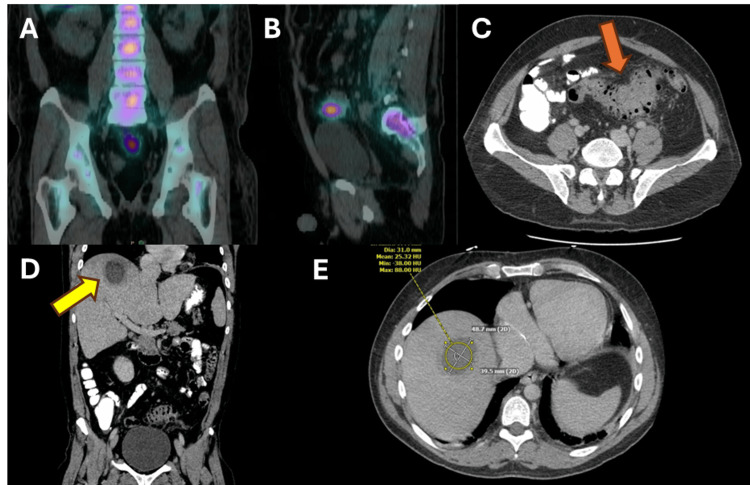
Imaging Studies of the Hepatic Abscess and Diverticulitis Indium-111 radionuclide scan demonstrating the area of rectosigmoid inflammation seen initially in coronal (A) and sagittal (B) views. When the patient re-presented, his computed tomography (CT) scan of the abdomen and pelvis demonstrated diverticulitis without frank abscess (red arrow) in the area previously highlighted on the radionuclide scan (C) and a 4 cm by 5 cm hepatic dome abscess (yellow arrow) seen in coronal (D) and transverse (E) views.
